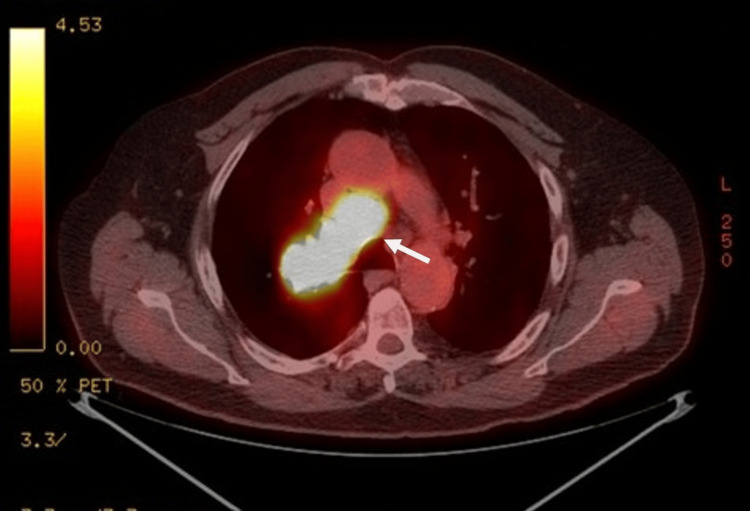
Axial PET/CT demonstrating hypermetabolic right hilar mass. An axial PET/CT image shows intense uptake (white arrow) in the right hilar region. PET-CT: positron emission tomography-computed tomography.
